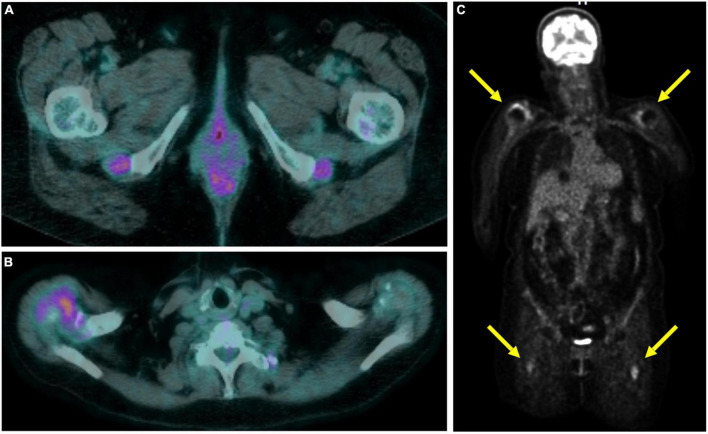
Total body 18F-fluorodeoxyglucose positron emission tomography-computed tomography (^18^F-FDG-PET/CT) in a polymyalgia rheumatica (PMR) patient with persistent articular inflammation in the ischial tuberosities (A) and right shoulder (B). Coronal slice in maximum intensity projection showing the uptake at the shoulders and subtrochanteric bursae (C).
